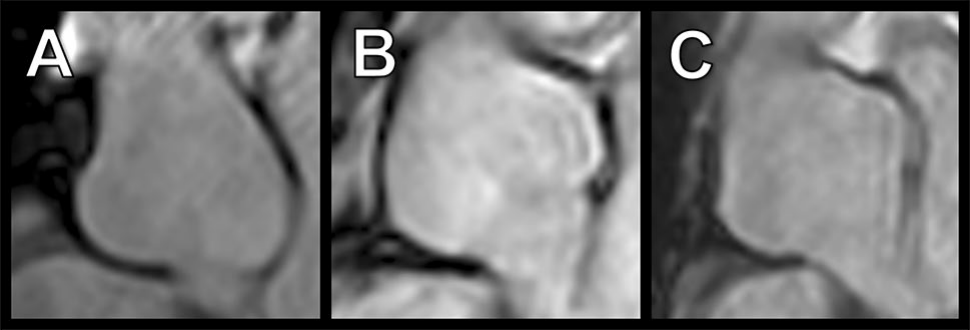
Para-sagittal non-contrast MRA of three Marfan patients with aortic root aneurysms with diameters larger than 45 mm. a–c All three aneurysms were correctly identified using MRA-derived inner-to-inner measurements. These findings were confirmed by echocardiography and all patients underwent prophylactic aortic root replacement. Both readers rated the inner vessel wall delineation for the sinuses of Valsalva as superior when compared to outer vessel wall delineation. Both observers measured comparable inner diameters: A: 47 vs. 48 mm; B: 51 vs. 51 mm; C: 50 vs. 50 mm which were similar to diameters obtained by echocardiography: A: 46 mm; B: 49 mm; C: 51 mm. Outer diameters showed a higher deviation: A: 50 vs. 52 mm B: 55 vs. 59 mm; C: 53 vs. 57 mm
